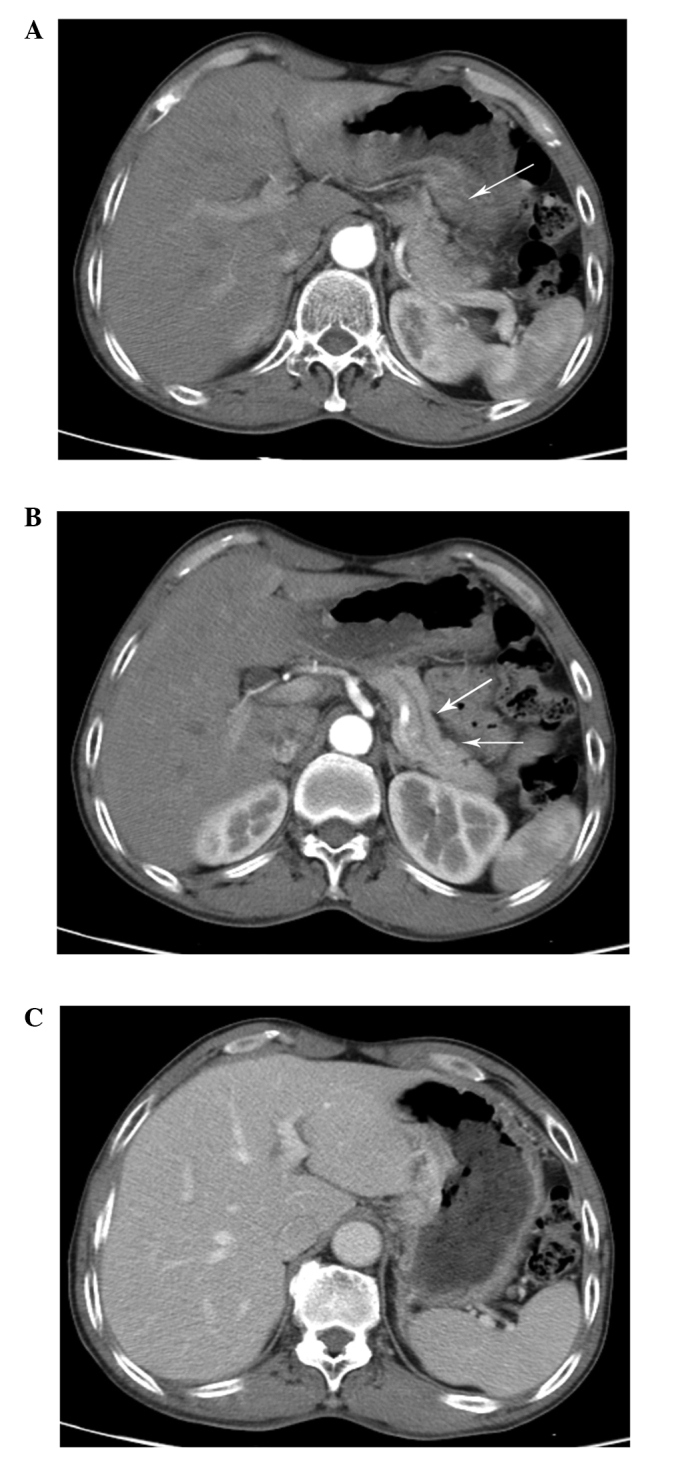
(A and B) Enhanced computed tomography following two cycles of neoadjuvant chemotherapy revealing that the lesion (arrows) was clearly decreased in size. (C) There is no evident nodule in the liver.
